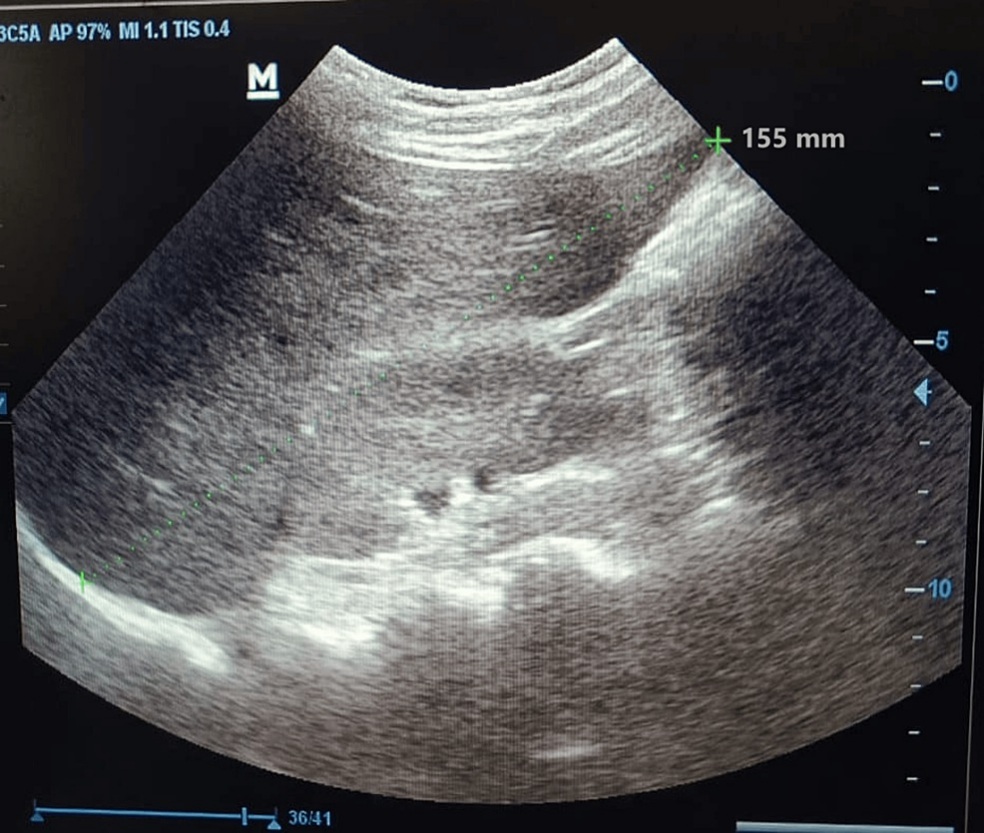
The image shows ultrasound of liver with a liver span of 155 mm indicating hepatomegaly. The dotted line (in green) represents the liver span equal to 155 mm.
